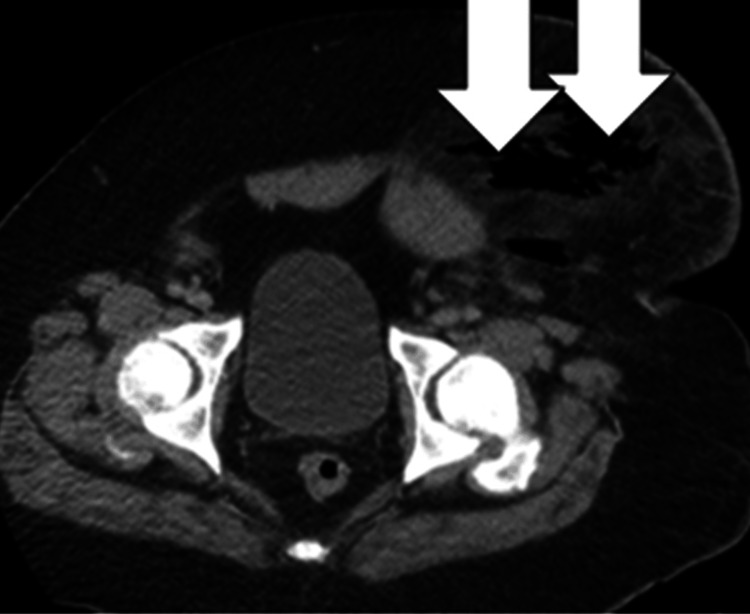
Evidence of subcutaneous gas in the left abdominal wall on CT scan in the Emergency Department
